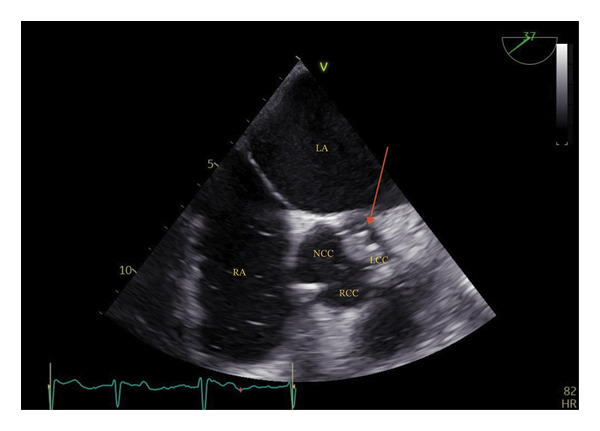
Transoesophageal echocardiography of the aortic valve showing a 10 × 10 mm vegetation located on the left coronary cusp. Red arrow indicates vegetation. Abbreviations: LA: left atrium, RA: right atrium, LCC: left coronary cusp, RCC: right coronary cusp, NCC: noncoronary cusp.
